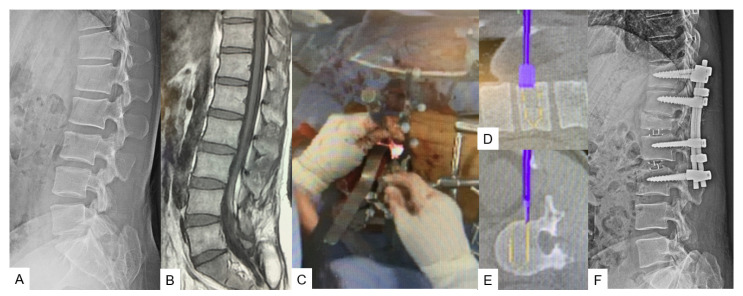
Case 5, 33-year-old male, Tethered cord syndrome, L1 shortening osteotomy with lateral osteotomy. (A) Preoperative lateral radiogram, (B) Preoperative T1 weighted MR imaging, (C) Intraoperative image, (D,E) Navigation monitor, (F) Preoperative lateral radiogram.
